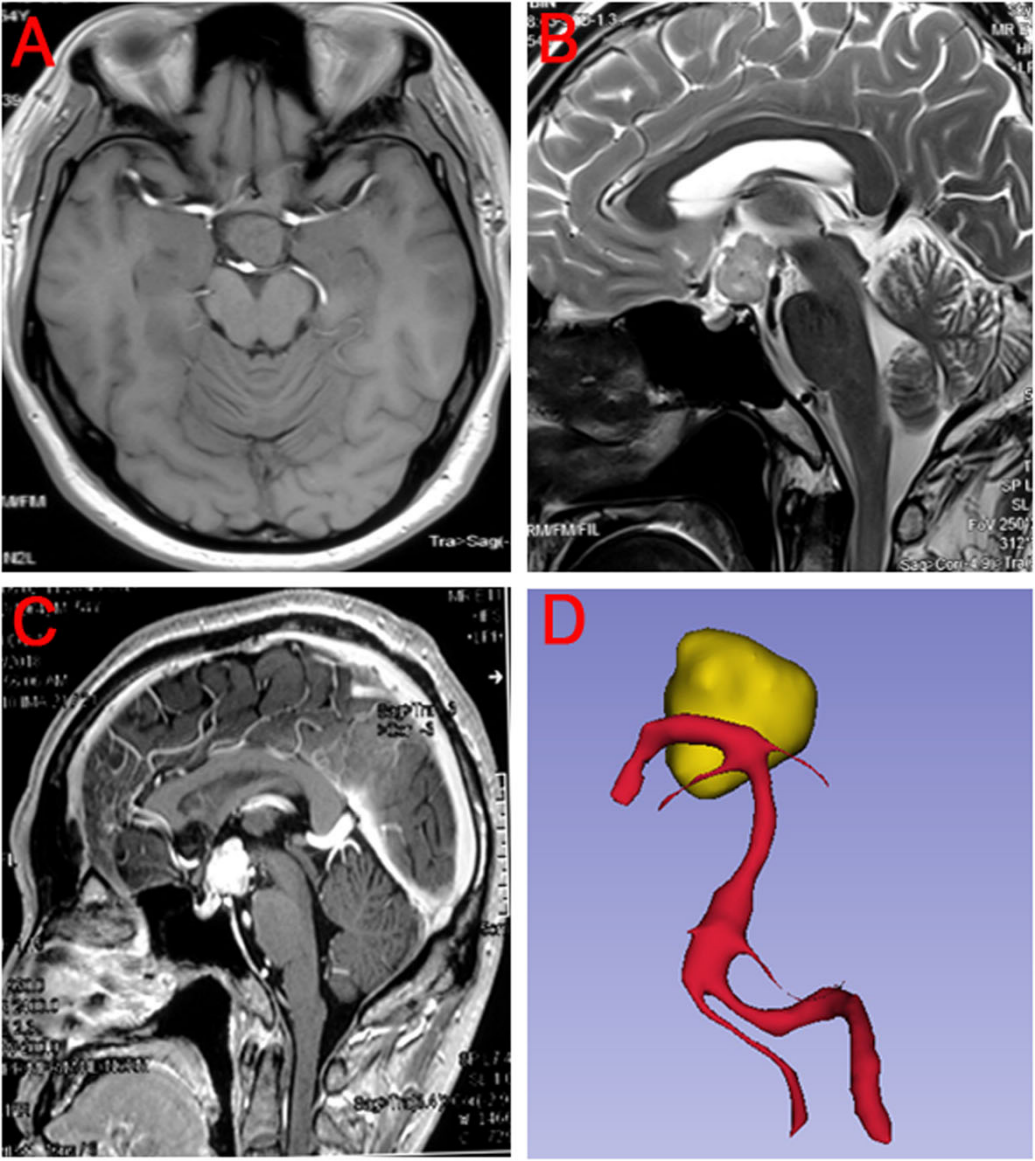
Preoperative brain MRI. a Axial T1WI MRI before the first operation showed an isointense lesion located at suprasellar region which was regular in shape and near the BA apex and PCA. b Sagittal T2WI MRI showed the lesion was isointense and closely related to the third ventricle. Moreover, there was no abnormity of BA. c Sagittal contrast-enhanced T1WI MRI showed the tumor was homogeneously enhanced and the BA was also shown. d Three dimensional-reconstructed image from preoperative MRI showed no aneurysm at BA and its branches
